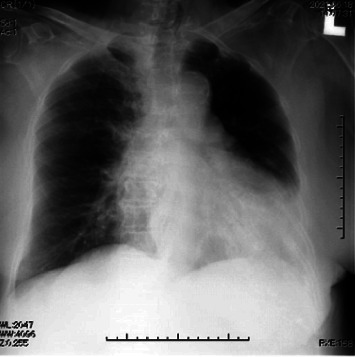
Chest radiograph on postoperative day 1.
